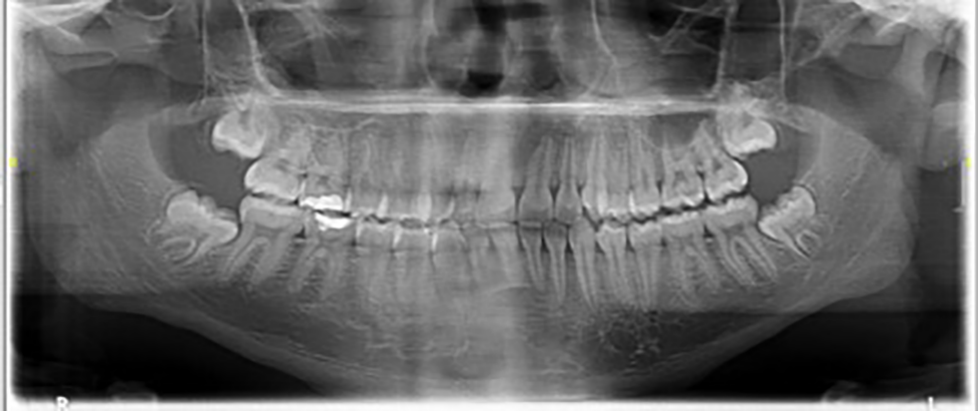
Panoramic radiograph demonstrating large restoration of tooth 3 with periapical radiolucency and right maxillary sinusitis.
